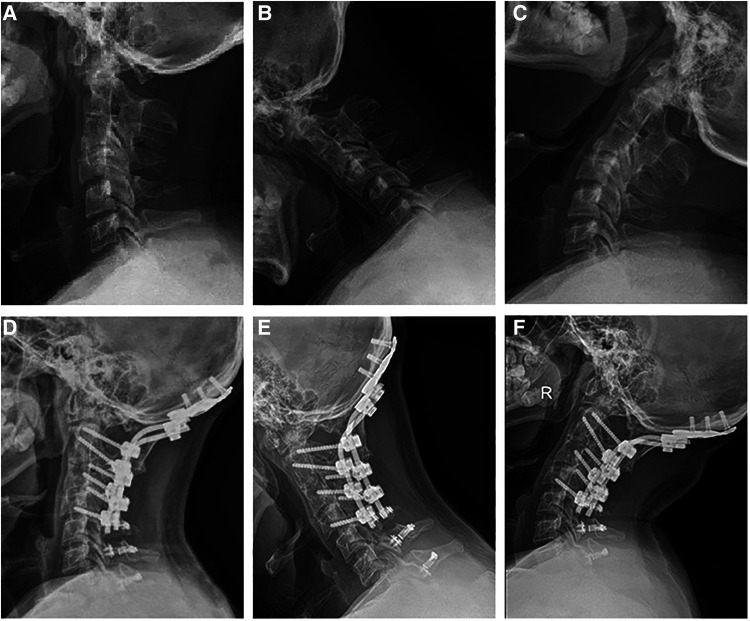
A 41-year-old male underwent a hybrid surgery for combined posterior occipitocervical fusion and subaxial laminoplasty (C0–7). (A–C) Preoperative lateral radiographs showed that the C0–2 Cobb angle was 15.5°, C2–7 Cobb angle was 24.6°, and ROM was 54.3°. (D–F) Last follow-up lateral radiographs showed that the C0–2 Cobb angle was 17.2°, C2–7 Cobb angle was 24.2°, and ROM was 36.15°. ROM, range of motion.
